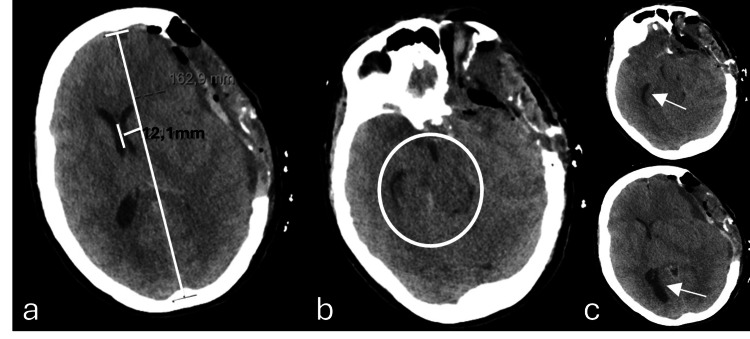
Axial plane of brain computed tomography taken 12 hours after post-craniectomy enlargement in the left temporoparietal region (third surgical intervention) due to neurological deterioration with fixed mydriasis There is evidence of bi-hemispheric convexity grooves effacement, base cisterns effacement, and 12 mm midline deviation to the right (a), as well as subfalcine herniation and inferior transtentorial herniation of the left uncus, with marked deformation of the midbrain (b). There is a slight increase in the amplitude of the temporal horn (superior arrow) and the occipital horn (inferior arrow) of the right lateral ventricle, reflecting an increase in internal CSF tension, due to disturbance of normal circulation (c).
